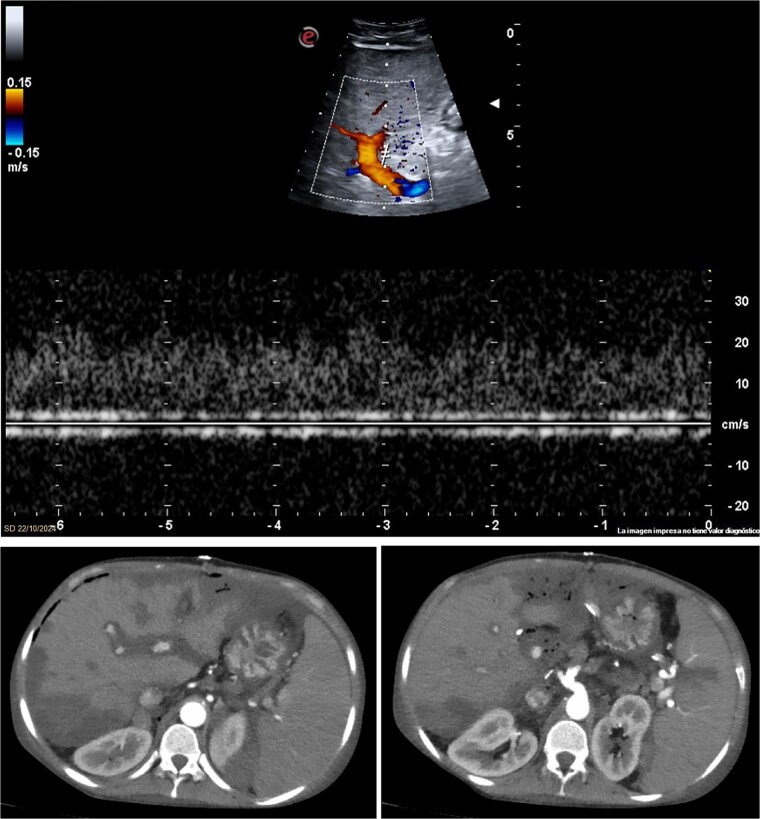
Imaging studies confirming hepatic artery thrombosis. (a) Doppler ultrasound showing absent hepatic artery flow. (b and c) CT angiography showing proximal hepatic artery occlusion with multiple hypodense areas in the liver parenchyma, consistent with ischemia and infarction.
